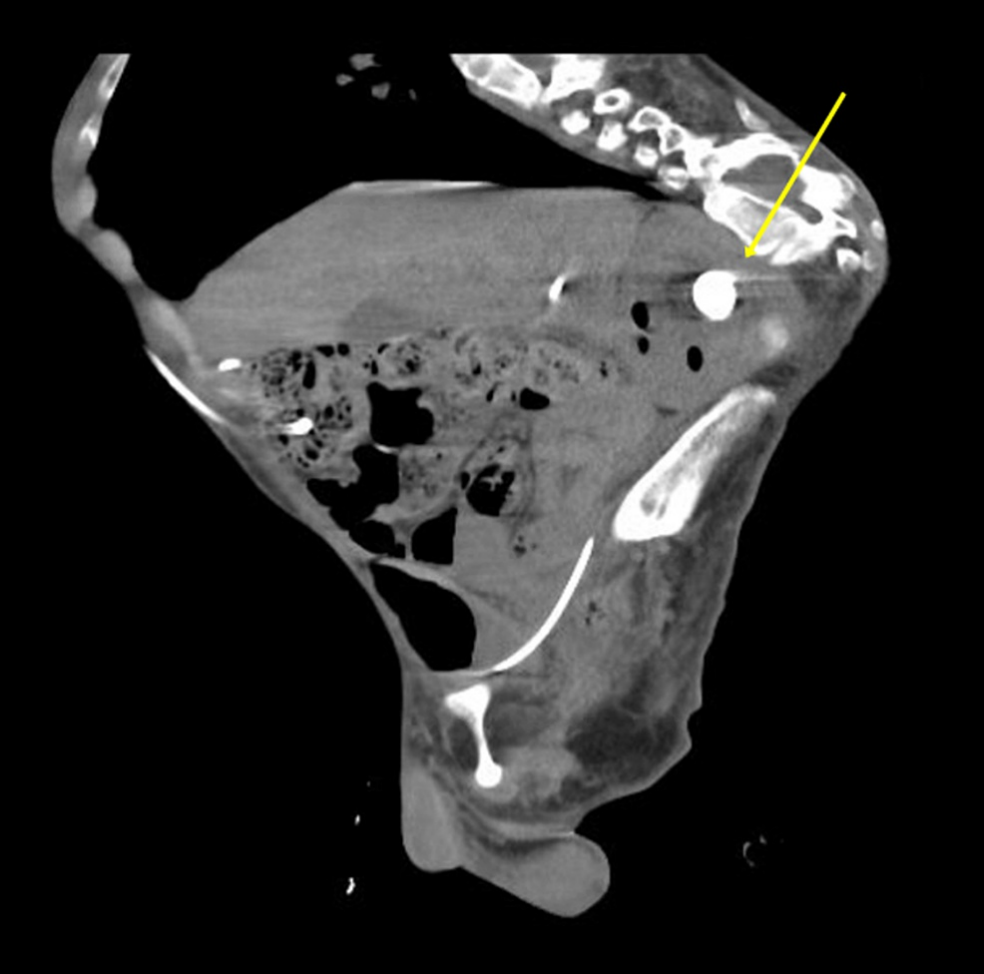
A sagittal view of the CT scan showing a radiopaque staghorn stone (yellow arrow) in a malrotated kidney with mild hydronephrosis. CT: computed tomography.
